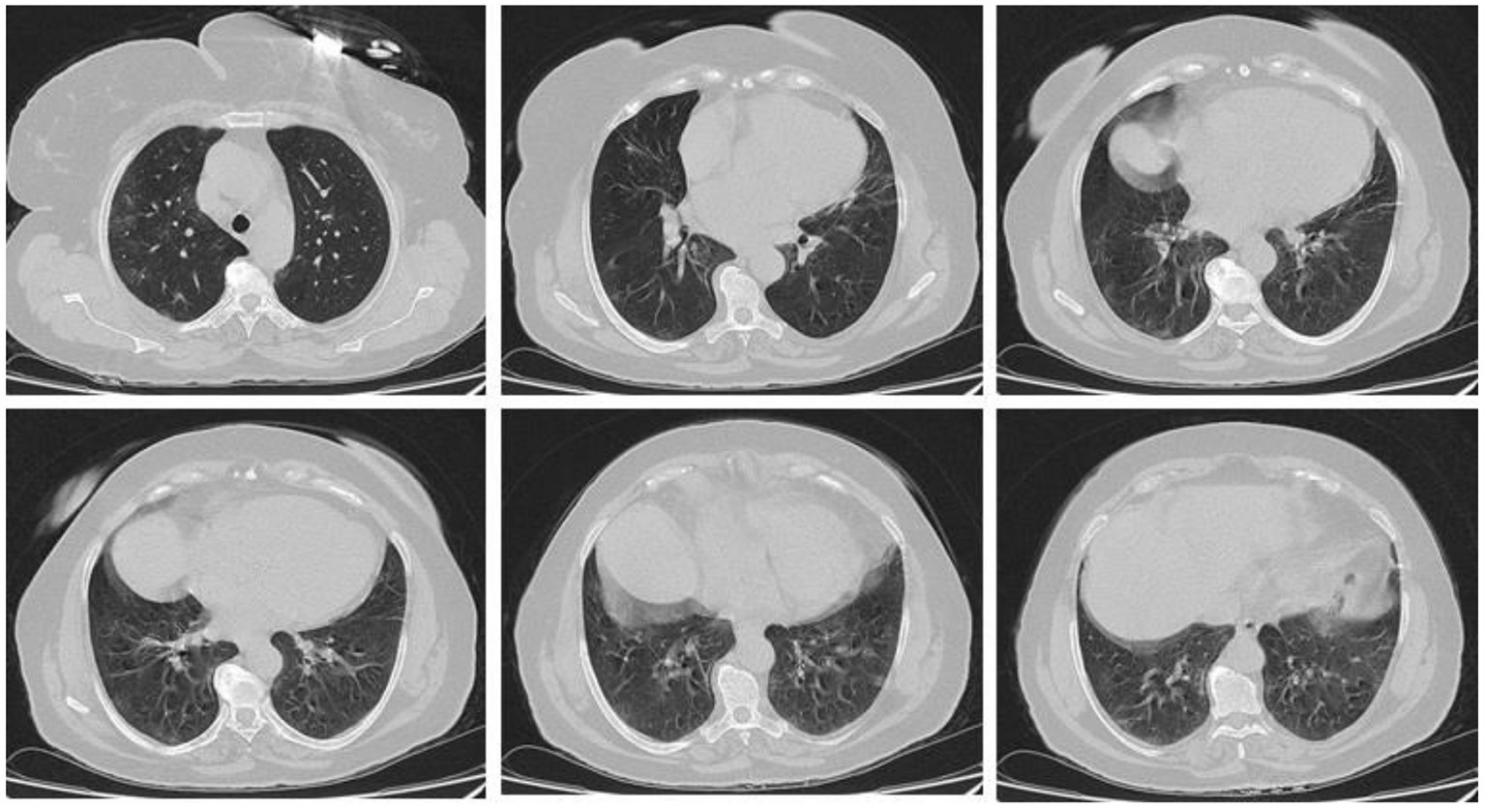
High resolution, axial non-enhanced spiral chest CT images (lung window) of a 59-years old patient who was confirmed to be infected by COVID-19 and admitted to hospital with Flu-like symptoms and gastrointestinal tract symptoms (mostly diarrhea). CT images show multiple faint patchy consolidations disseminated in ground-glass patterns.
